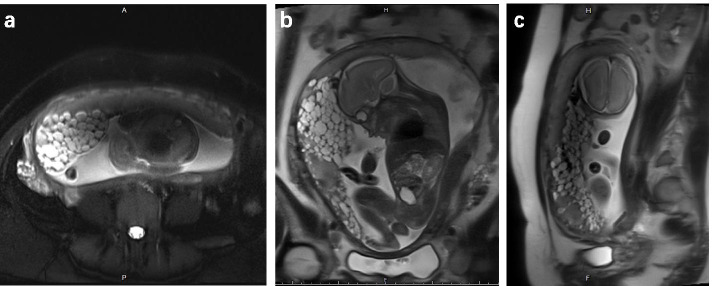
MR images displaying a placenta with a normally-appearing portion and an abutting multicystic mass with high signal on T2-weighted images at the right anterior wall. The mass is partly admixed with the normal placental parenchyma and partly bulged between the normal placental parenchyma and the amniotic sac
